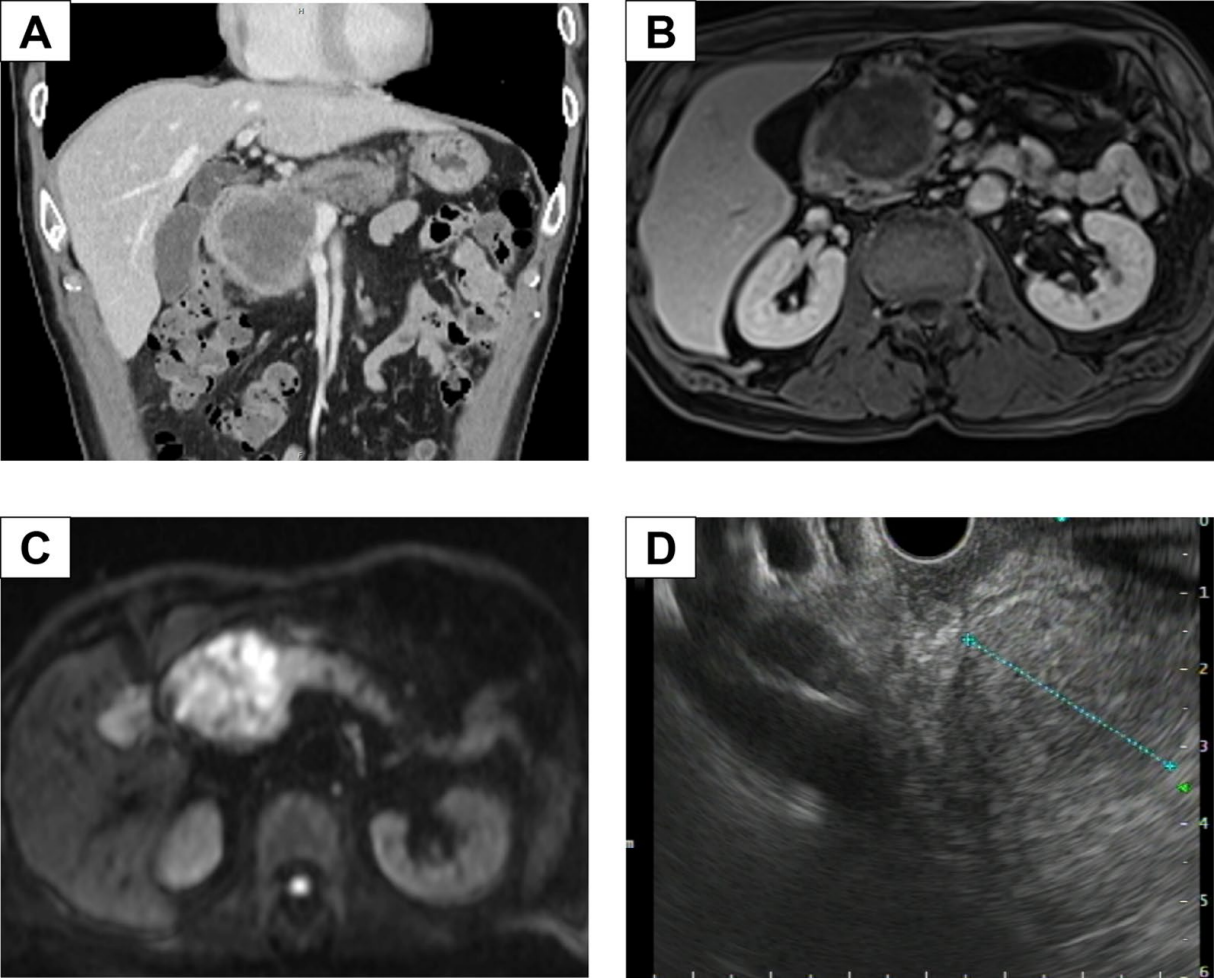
Preoperative images. A Abdominal CT in the coronal section revealed a well-demarcated cystic tumor with slight enhancement of the peripheral portion of the pancreatic head. B MRI revealed a pancreatic head tumor with SMV invasion. C Diffusion-weighted magnetic resonance imaging showing reduced tumor diffusion. D Diffusion-weighted images showing a pancreatic head mass with a high signal intensity
